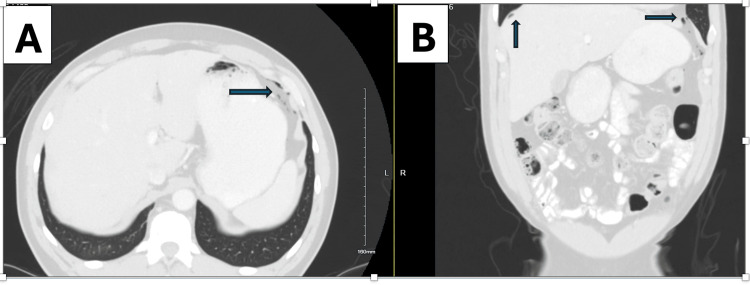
Axial view (A) and (B) coronal view CT scan images showing free intraperitoneal air in axial and sagittal views, arrows pointing at the free air CT: computed tomography
